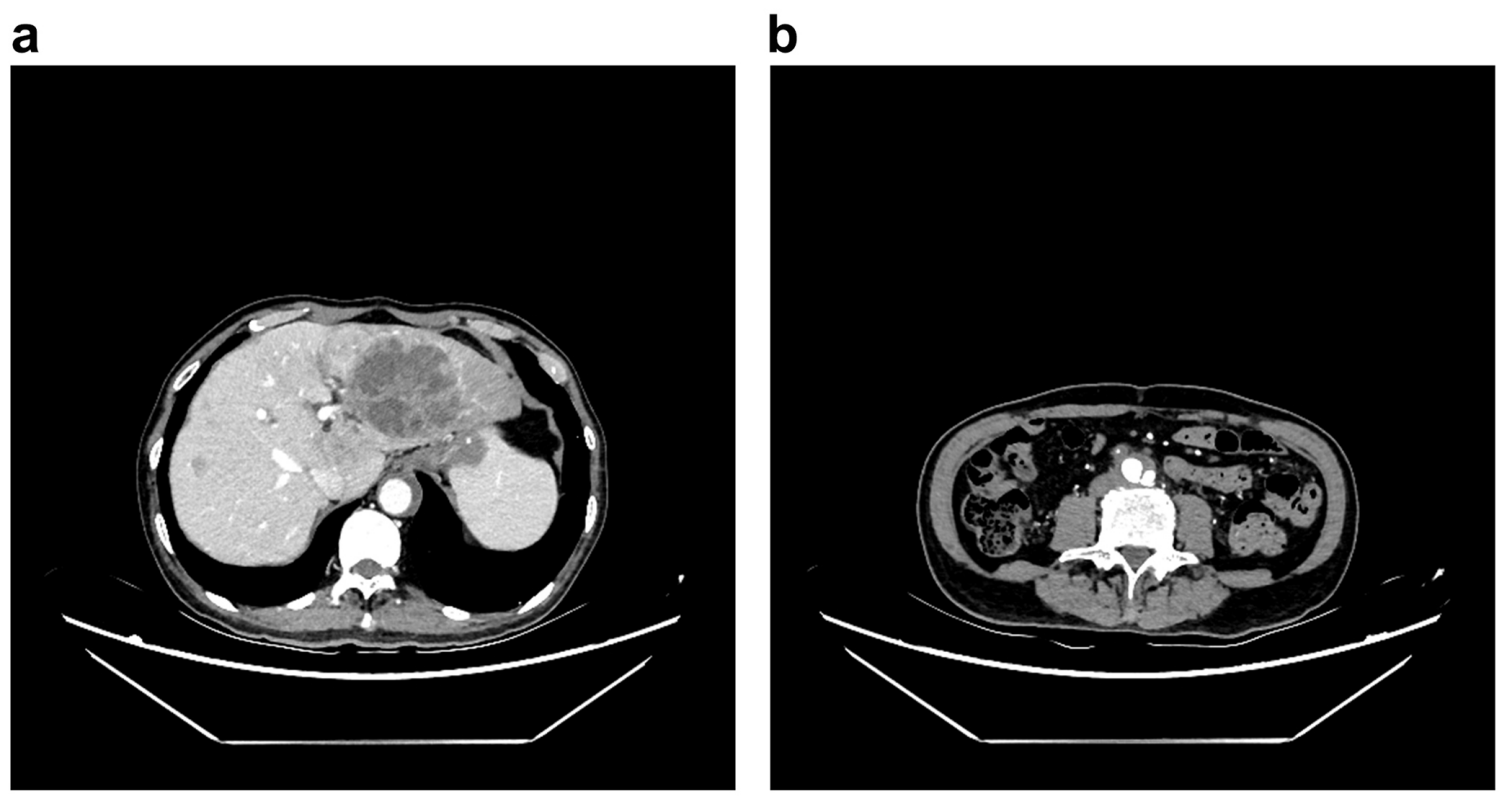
Signs of abdominal aortic dissection in the computed tomography examination, (a) abnormal outline of abdominal aorta in the level of liver, (b) outline of aortic dissection in the level of lower abdomen.
